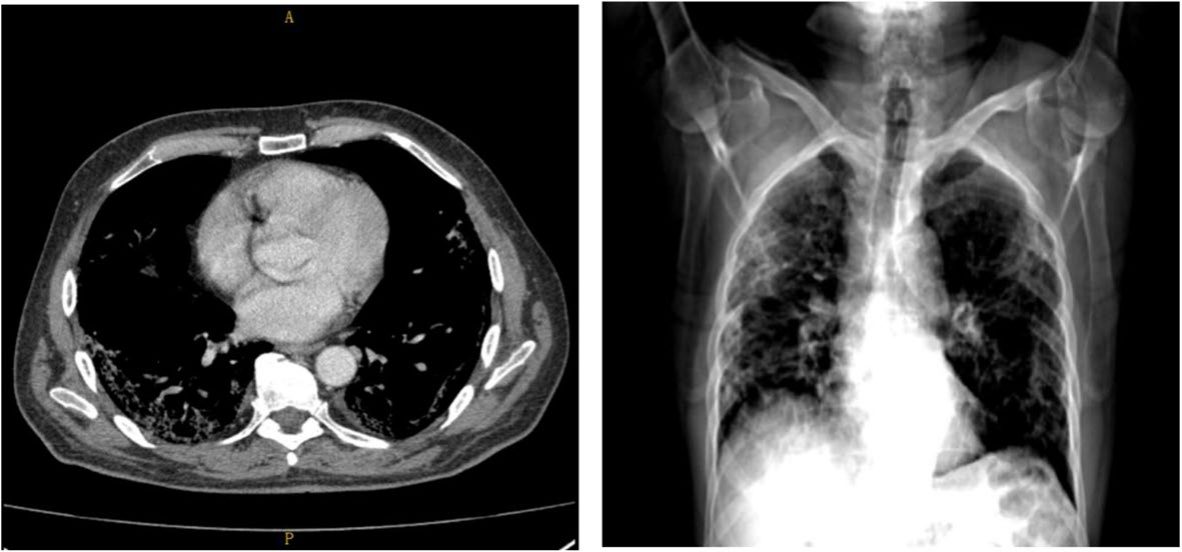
Spiral computed tomography of the chest shows irregular and advanced interstitial changes and ground-glass opacities in peripheral areas of lungs
